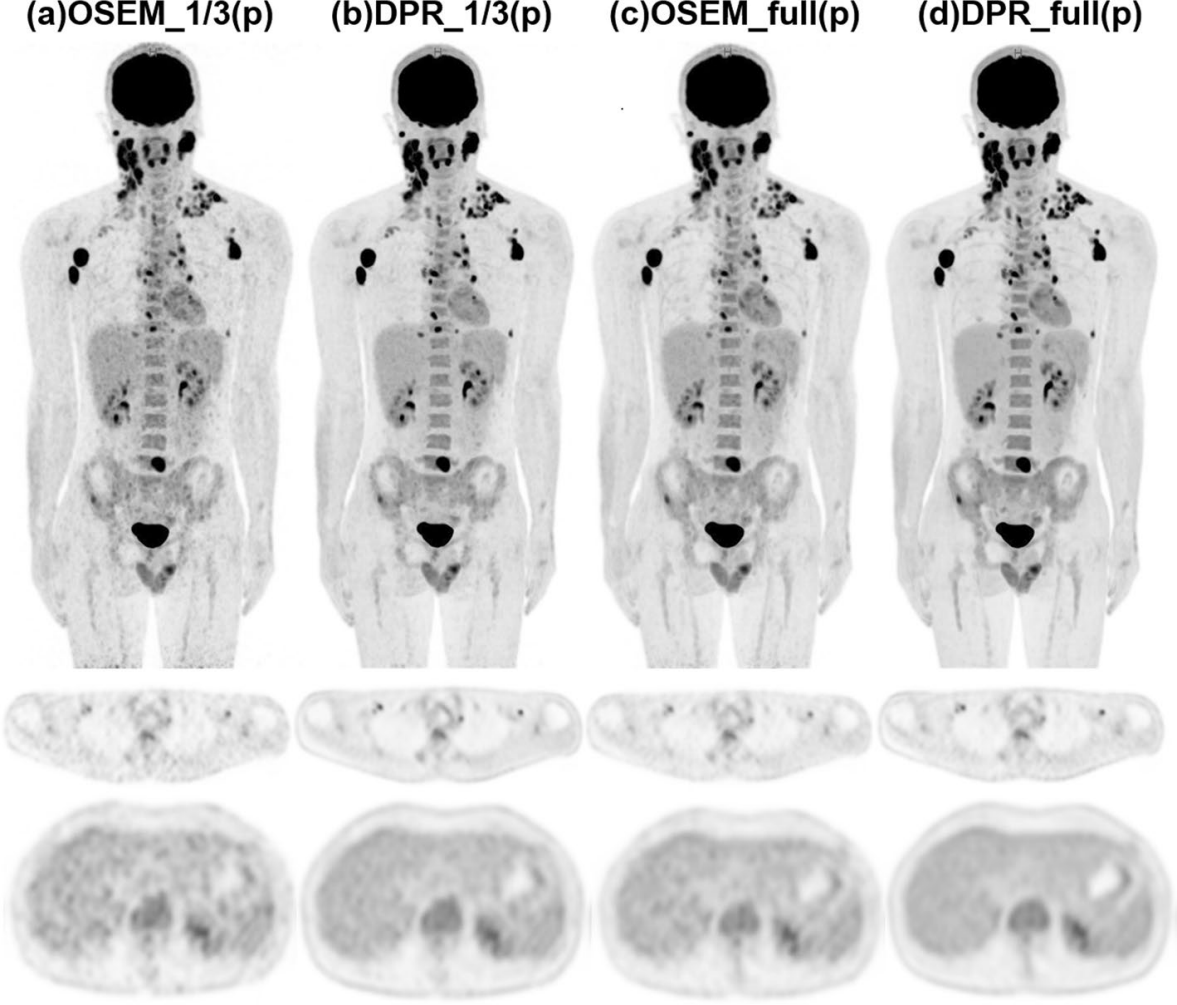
PET images of a 31-year-old male patient diagnosed with Hodgkin's lymphoma in the prospective study. After injection of 85 MBq ^18^F-FDG, the patient underwent a PET scan with 6 min per bed position 77 min post-injection. To comprehensively compare the clinical images reconstructed by the two algorithms, we further reconstructed the data with 1/3 and full of the acquisition duration for OSEM and DPR algorithms. Both the MIP (upper row) and transverse images (middle row) demonstrated improved lesion detectability of the DPR images compared to the OSEM images with the same acquisition duration (a–d). The transverse image in the liver plane showed reduced image noise when comparing subfigures a–d, while the image noise in the subfigures b and c was at a comparable level
